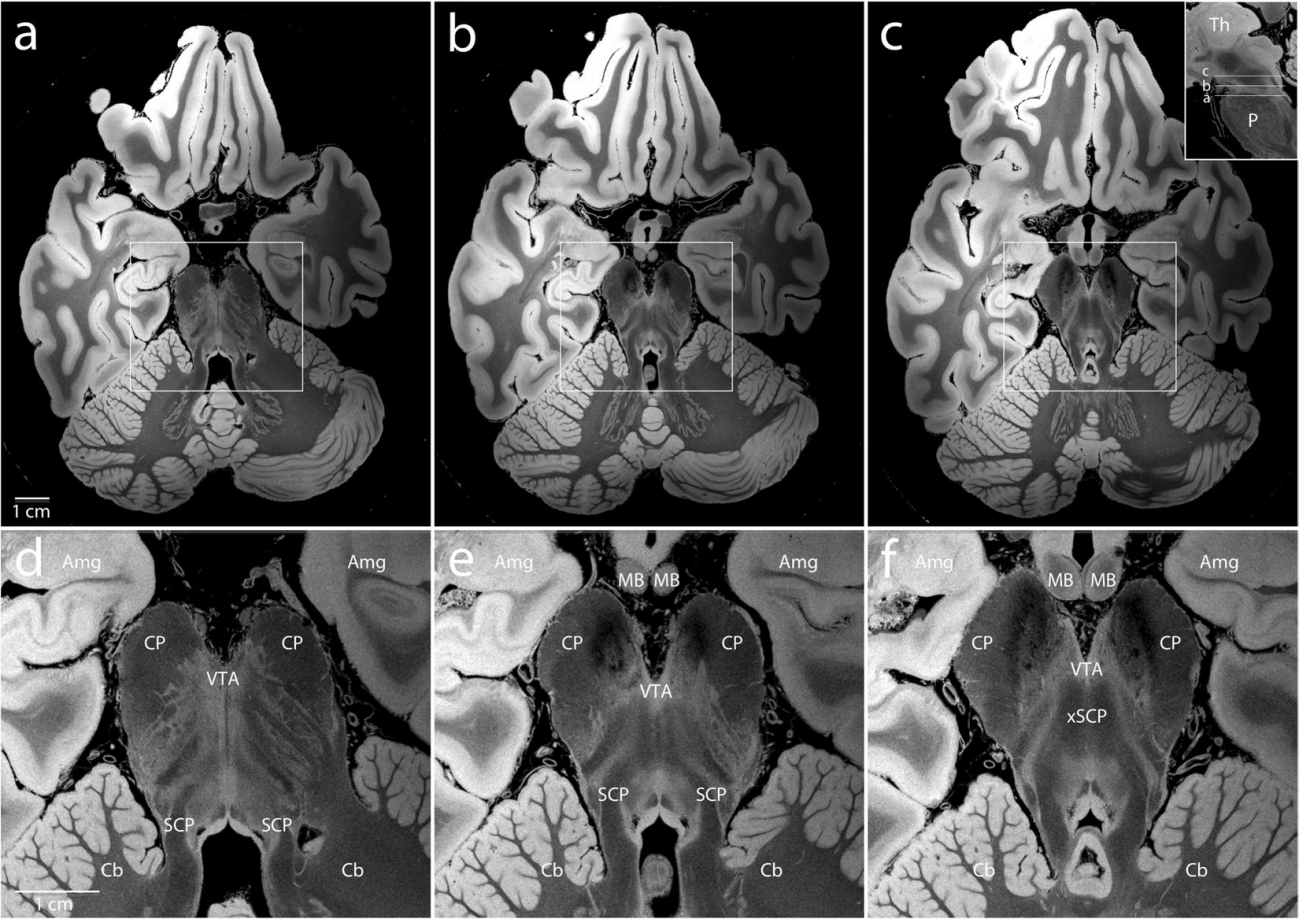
Delineation of brainstem neuroanatomy. Representative axial sections from the synthesized FLASH25 volume are shown at the level of the rostral pons and caudal midbrain (a-c, see inset in panel c). Zoomed views of the brainstem, medial temporal lobe, and anterior cerebellum (within the white rectangles in a–c) are shown in the bottom row (d–f). The anatomic detail that can be visualized in this *ex vivo* 100 μm resolution MRI dataset is beyond that which can be seen in typical *in vivo* MRI datasets. All images are shown in radiologic convention. Neuroanatomic abbreviations: Amg = amygdala; Cb = cerebellum; CP = cerebral peduncle; MB = mammillary body; P = pons; SCP = superior cerebellar peduncle; VTA = ventral tegmental area; xSCP = decussation of the superior cerebellar peduncle; Th = thalamus.
